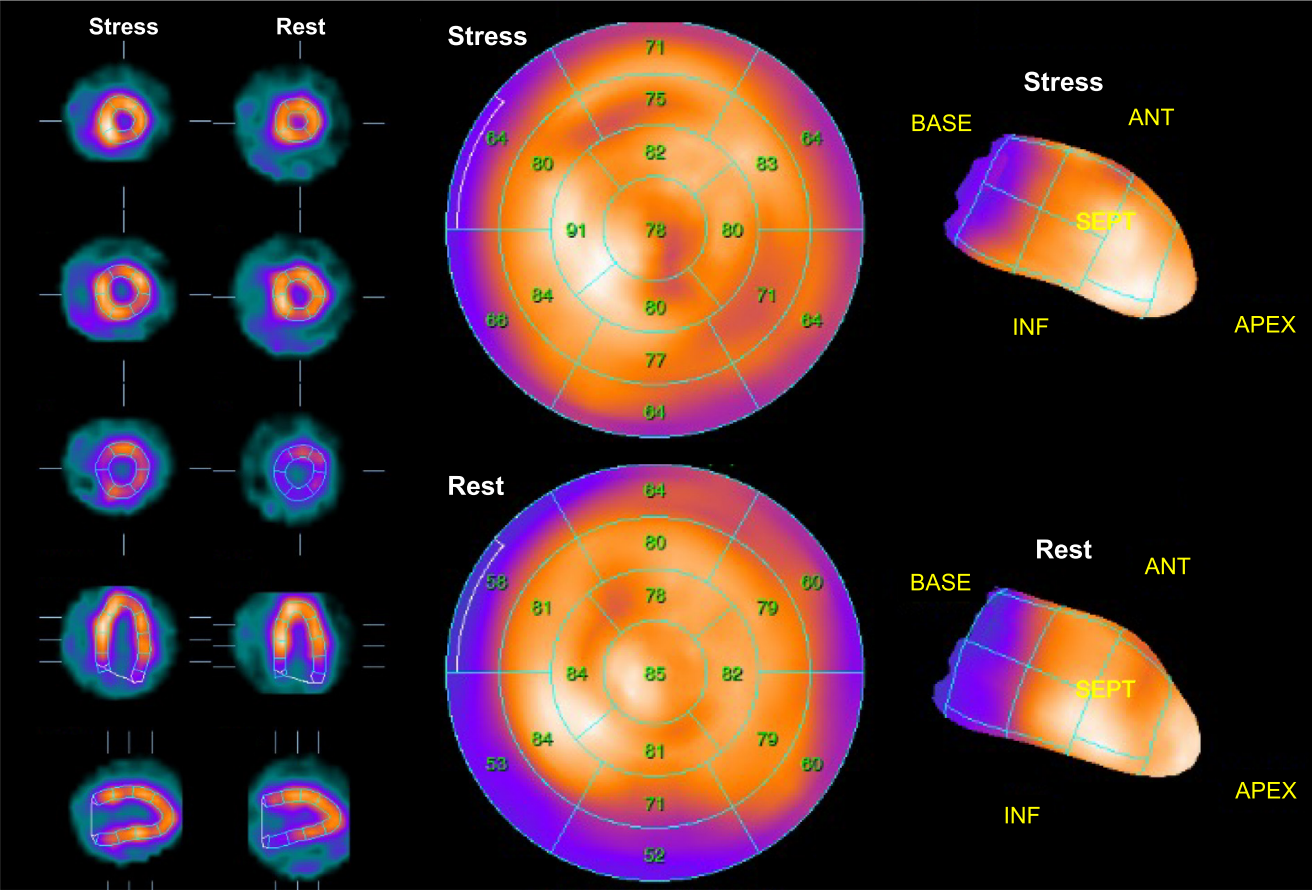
Adenosine stress thallium-201 myocardial perfusion scintigraphy. A normal perfusion pattern was observed (summed stress score = 3; summed rest score = 0; summed difference score = 3; stress defect = 4 %; transient ischemic dilatation ratio = 1.20).
